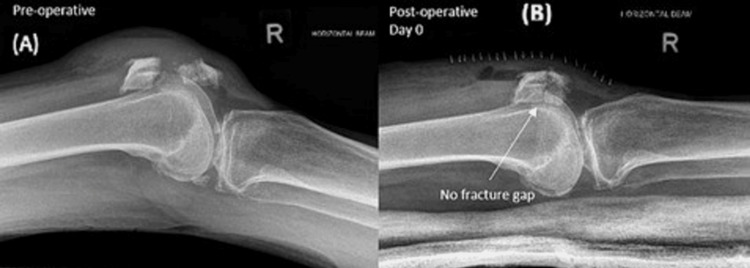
Radiograph of a mid-pole transverse patellar fracture fixed with the all-suture technique (A) Preoperative X-ray showing the mid-pole displaced transverse patellar fracture (B) Postoperative X-ray after suture fixation of the patellar fracture
